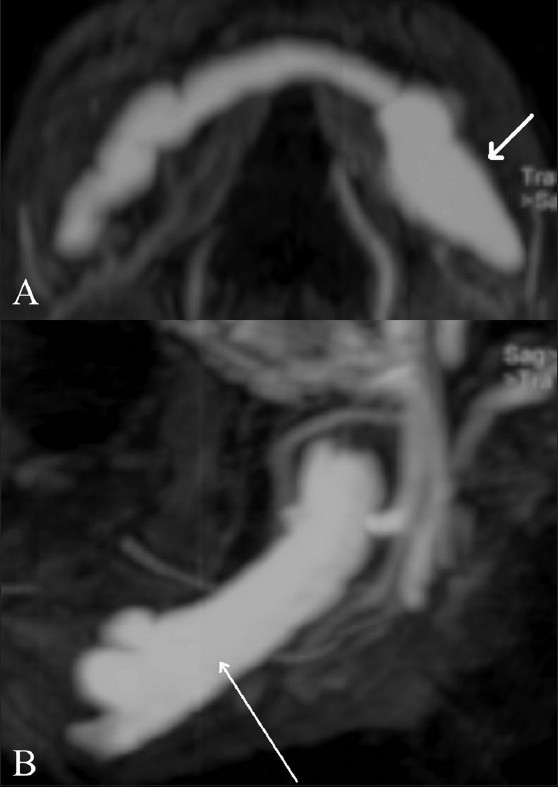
Axial (A) and sagittal (B) contrast enhanced T1W MR images show the arteriovenous malformation (AVM) (arrows) involving the whole of the body of the mandible
